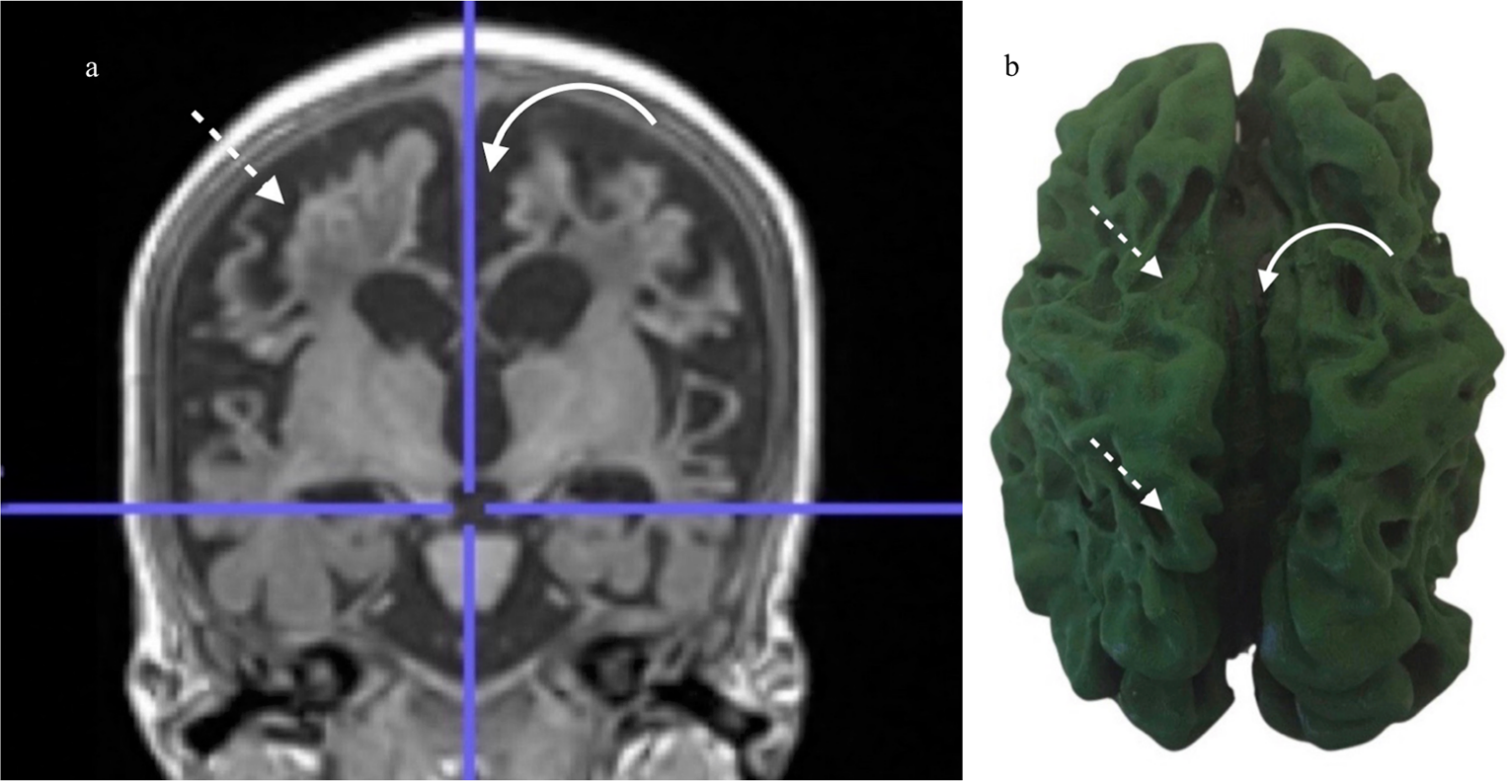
a, b Severe partial-prolonged hypoxic ischaemic injury to the brain with MR imaging at the age of 4 years and accompanying 3D print. a Coronal T1 weighted MRI at the level of the 3rd ventricle in a child with changes of severe term partial-prolonged hypoxic ischaemic injury, demonstrating marked widening of the interhemispheric fissure (curved arrow) with severe bilateral, symmetric volume loss in the anterior watershed distribution as well as perisylvian regions (dashed arrow). b 3D printed model of the brain shown in Fig. 3a demonstrating the pronounced interhemispheric and sulcal widening due to the volume loss (curved arrow) and severe bilateral, symmetric shrinkage of the brain along the peri-Sylvian and peri-Rolandic regions (dashed arrows)
